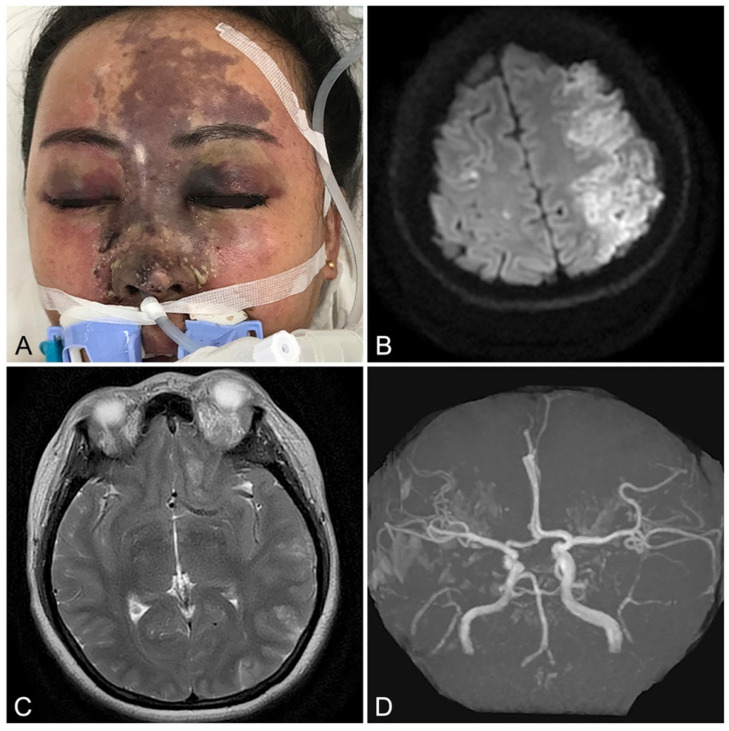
Example of extensive facial soft tissue ischemia with neuro-ophthalmological sequelae resulting from iatrogenic filler embolization: (A) Appearance of 40-year-old female 5 days following arterio-embolic injury resulting from dorsal nasal hyaluronic acid filler augmentation, revealing ischemic changes of the frontonasal skin of the face. (B) Diffusion-weighted axial magnetic resonance imaging (MRI) demonstrating left hemi-frontoparietal cerebral infarction. (C) T2-weighted axial MRI revealing edema and ischemic changes affecting the left optic nerve and orbital soft tissues. (D) Magnetic resonance angiography demonstrating intact proximal cerebral circulation (circle of Willis and anterior, middle, and posterior cerebral arteries), suggesting a distal embolic etiology. Reproduced with permission from Yang et al. [3], 2020, Springer Nature.
